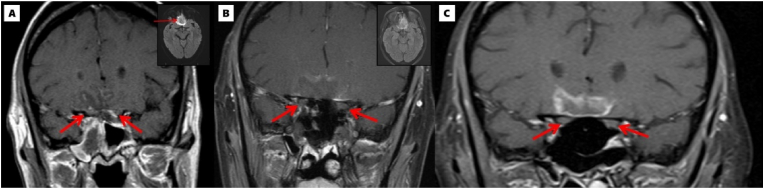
A) Brain MRI with GAD on presentation demonstrates corresponding restricted diffusion (inset) and leptomeningeal enhancement and regional sulcal effacement of the bilateral medial inferior frontal lobes including the gyrus recti. Mild dural thickening and enhancement in bilateral anterior cranial fossa were present. Red arrows showing optic nerves. B) Orbit MRI with GAD one day after right medial orbital, right optic nerve, and multi-sinus decompression demonstrates a similar appearing high FLAIR signal (inset) within the bilateral inferior frontal lobes including the gyrus recti with persistent restricted diffusion and leptomeningeal enhancement. Red arrows showing optic nerves. C) Orbit MRI with GAD after IV methylprednisolone for 3 days followed by prednisone 60 mg three times a day for 10 days demonstrates a significant decrease in the size of the area of abnormal signal involving bilateral inferior medial frontal lobes. Red arrows showing optic nerves. (For interpretation of the references to color in this figure legend, the reader is referred to the Web version of this article.)
